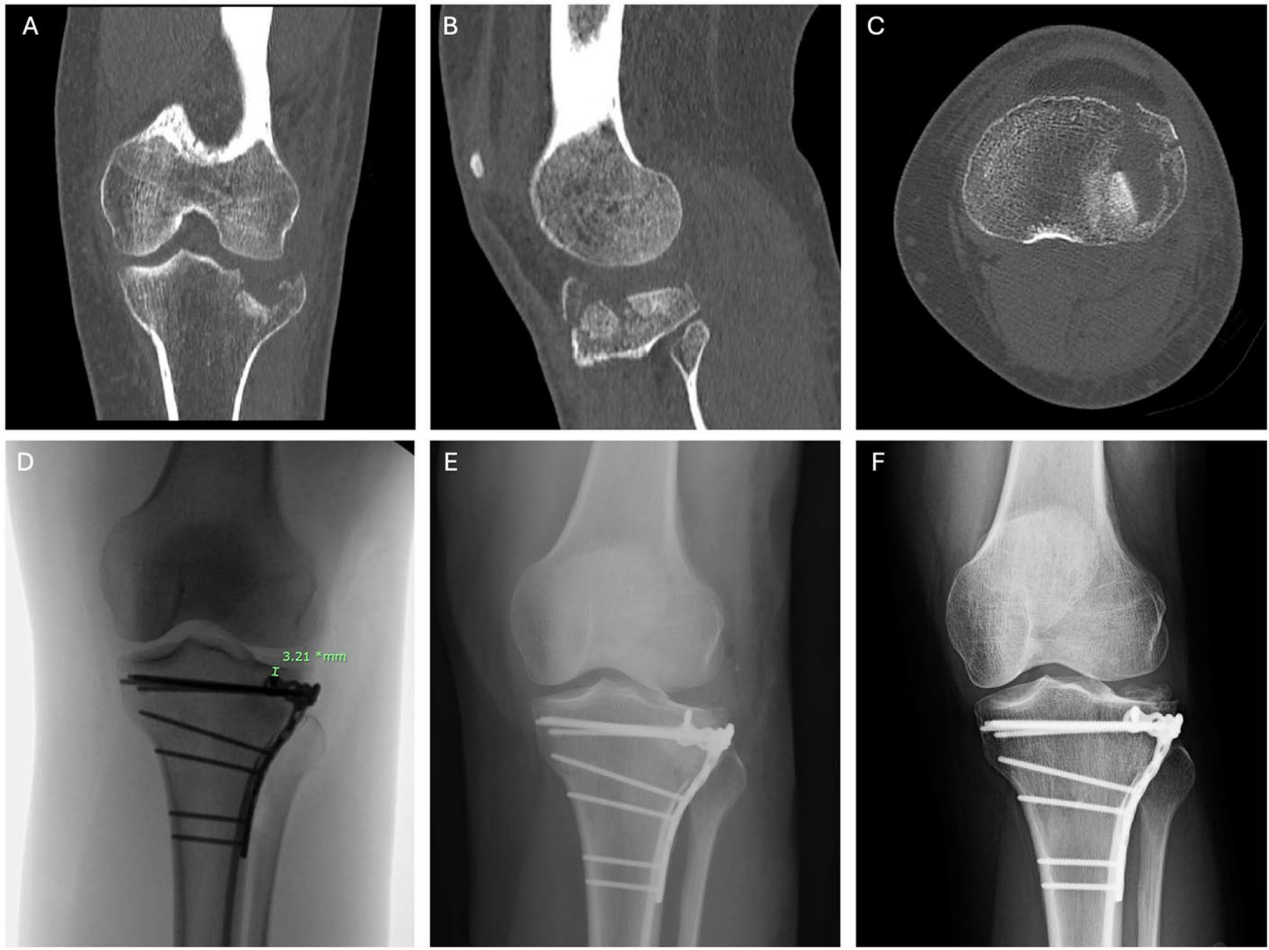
A 42-year-old female fell down stairs and received a 2.7-mm MF T-plate. Preoperative computed tomography demonstrates a left tibial plateau lateral split-depression fracture in the a coronal, b sagittal, and c axial planes. d The SJD measured on intraoperative fluoroscopy is 3.21 mm. e Immediately postoperatively. f Final follow-up at 119 weeks postoperatively shows maintenance of articular healing
